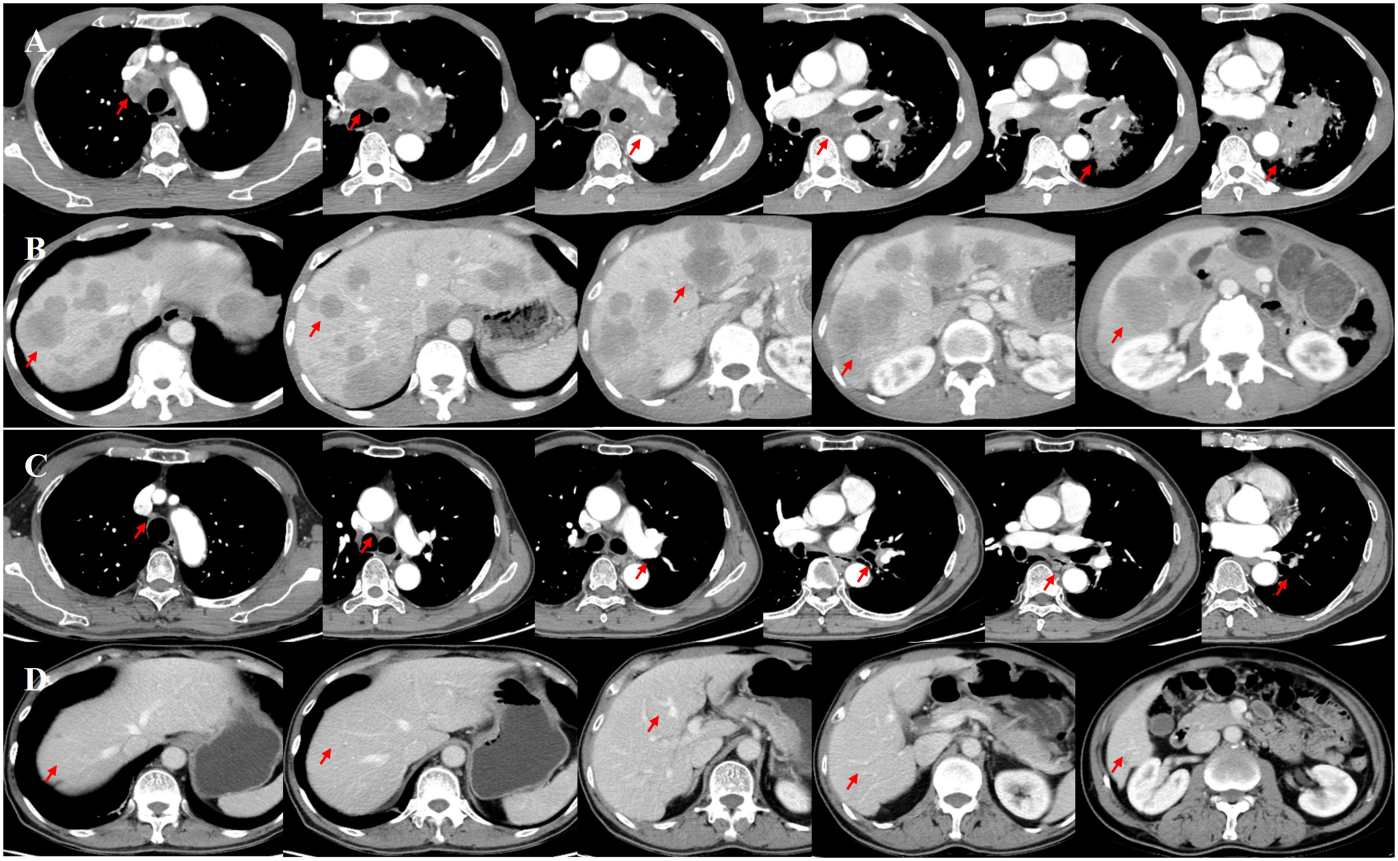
Enhanced computed tomography (CT) of the chest and abdomen was initially performed on December 27, 2022 (A, B). After four cycles of immunochemotherapy, a follow-up enhanced CT was performed on April 22, 2023, showing the disappearance of the primary lesion in the left lung, notable shrinkage of the left lung hilar and mediastinal lymph nodes (short axis <10 mm), and absence of obvious metastatic lesions in the liver (C, D).
